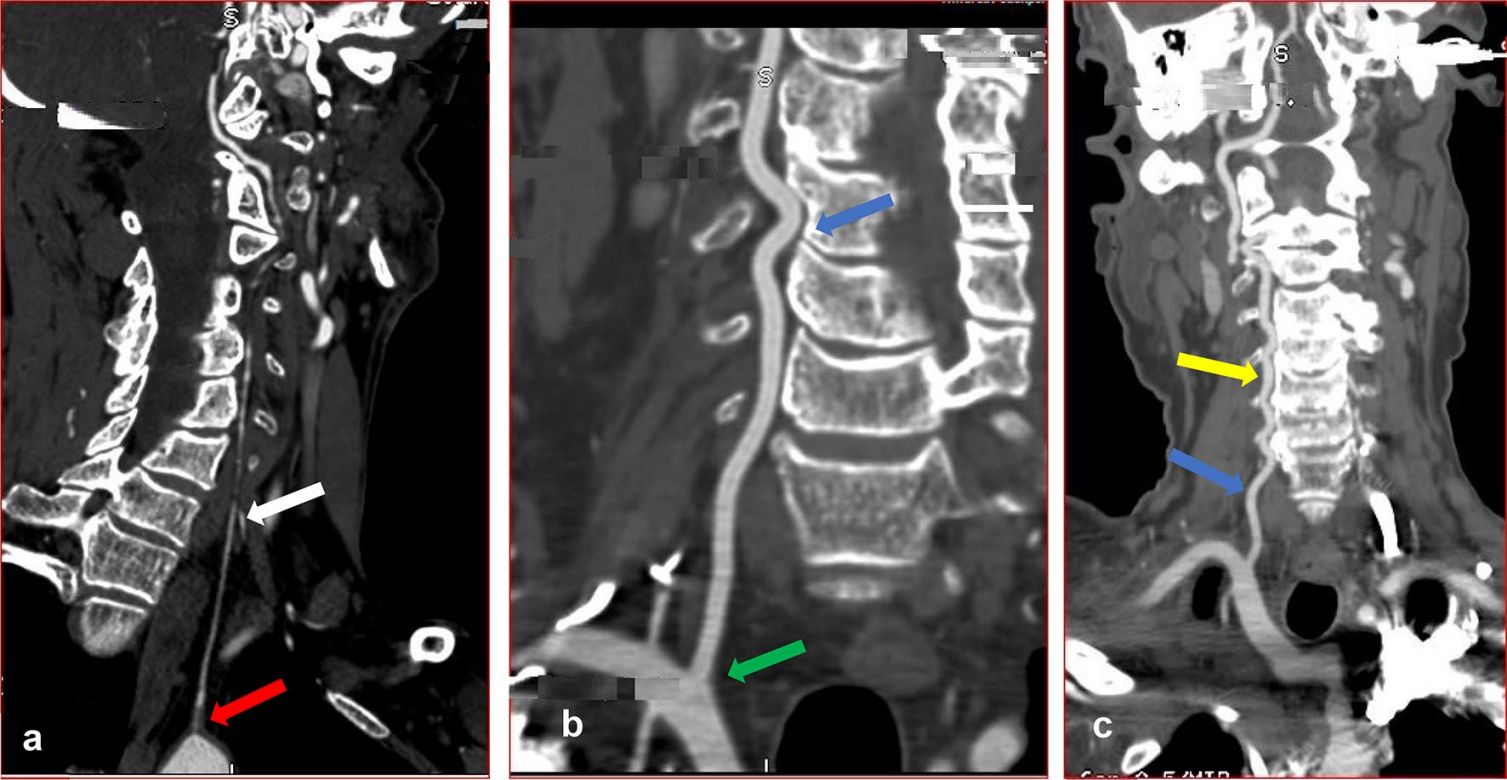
Coronal view of CTA images of a male (a, b) and female (c) patients showing variation in origin, hypoplasia, medial loop at V2 and mild tortuosity at V1 and V2. a Red arrow shows origin of the left hypoplastic vertebral artery (white arrow) from the aortic arch. b The dominant right VA emanates from the subclavian artery (green arrow) with a single medial loop into the vertebral body of C4 (blue arrow). c Shows mild tortuosity at V1(blue arrow) and V2 segments (yellow arrow) of the right VA (color figure online)
